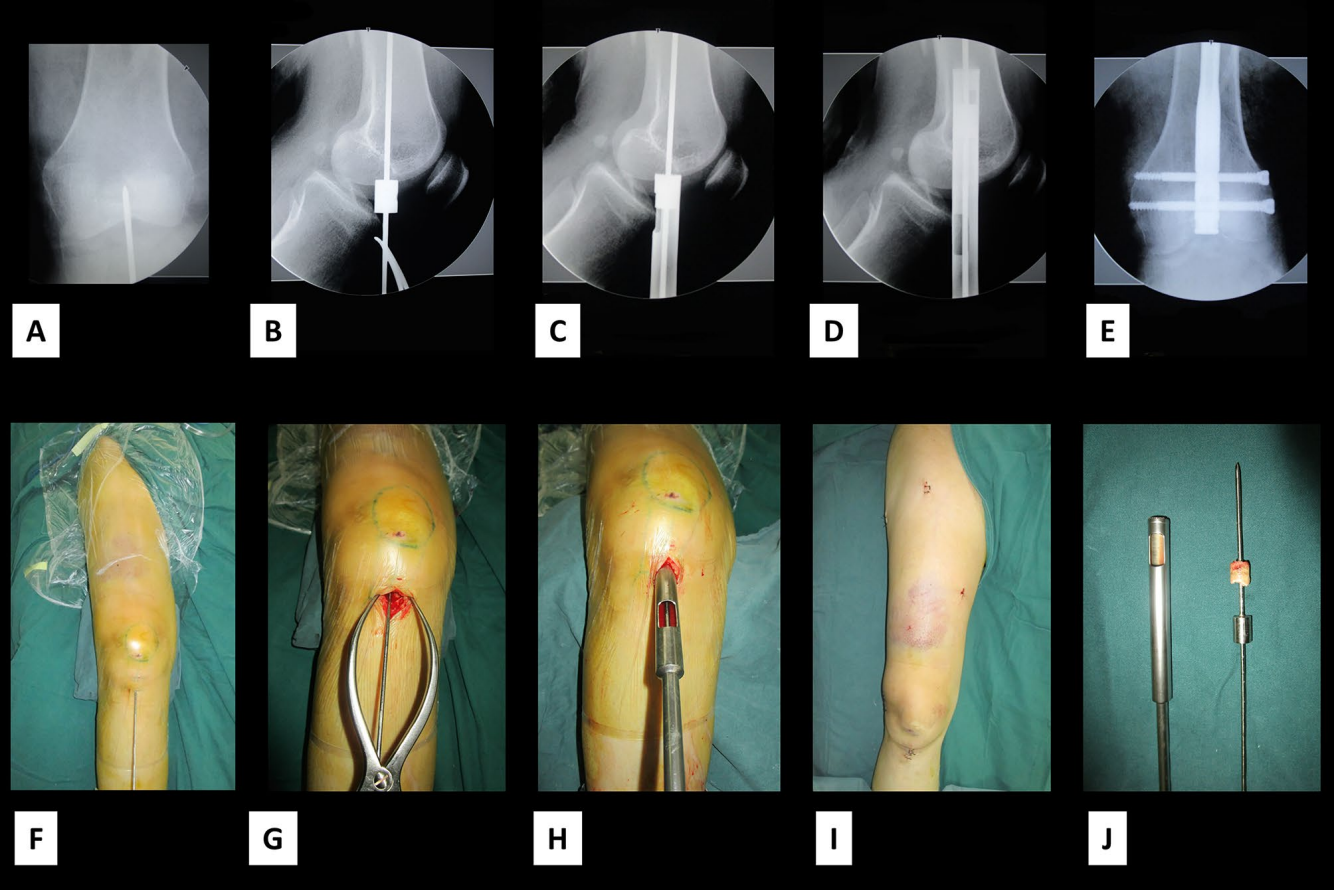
A-E: Intraoperative anteroposterior and lateral radiographs show the application of a hollow trephine F-I: The incision J: The hollow trephine and harvested bone bolt
